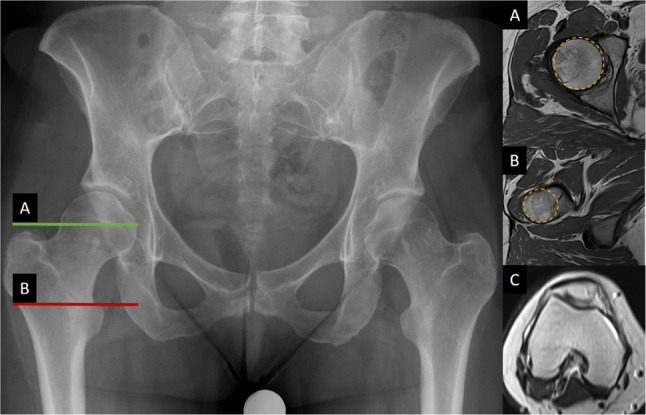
Measurement of femoral version on MRI according to the Murphy method. Representative patient case. A Femoral head center B base of the femoral neck C condylar axis
